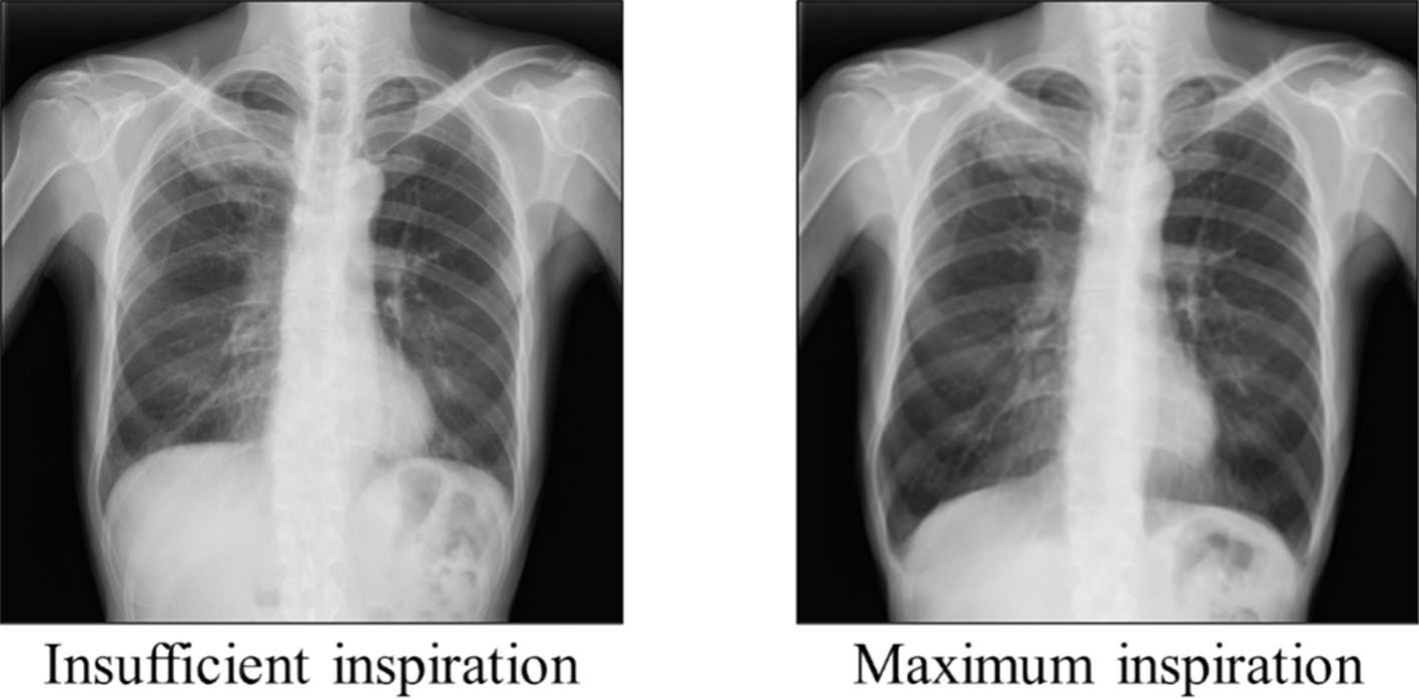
Chest X-ray images of pulmonary emphysema
